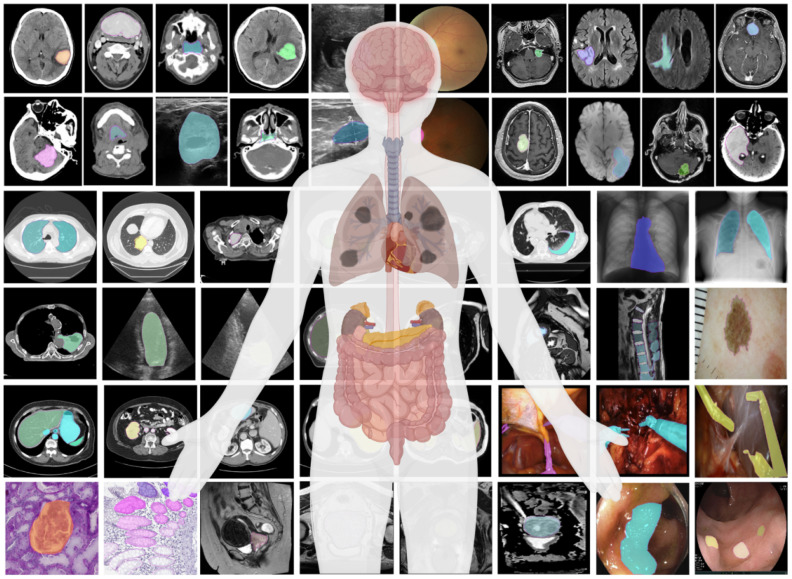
Overview of medical image segmentation tasks across diverse imaging modalities. The figure demonstrates the capability of modern foundation models (e.g., MedSAM [9]) to handle a variety of anatomical structures and pathological conditions in CT, MRI, and other modalities. Reproduced from [9] under the Creative Commons Attribution (CC BY 4.0) license.
